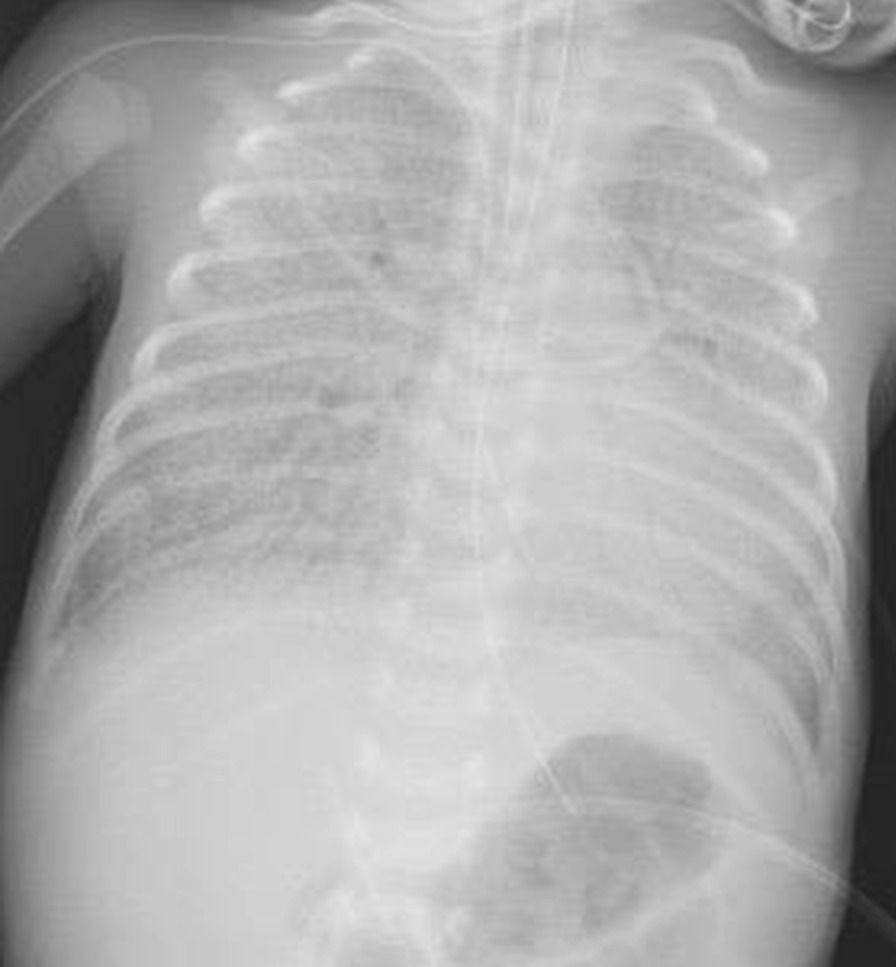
Patient’s chest X-ray during his early course of treatment
